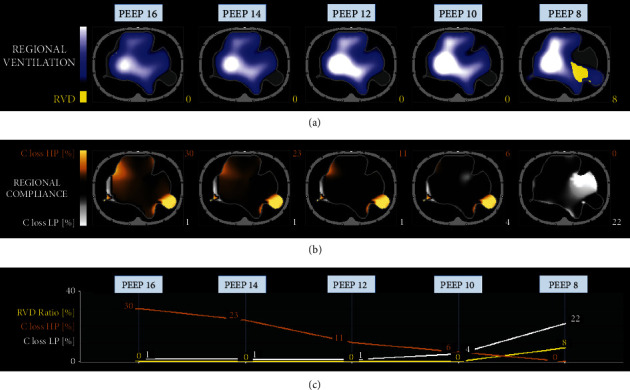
Electrical impedance tomography decremental positive end-expiratory pressure (PEEP) trial during the first extracorporeal membrane oxygenation support. In (a), the regional distribution of tidal ventilation at different PEEP values is shown by the blue–white gradient area, while the yellow area represents regional ventilation delay (RVD), whose percentage is indicated by the yellow number. In (b), regional compliance (C) is analyzed at different PEEP values. Compliance loss secondary to high PEEP (HP) is represented by the orange area (i.e., lung overdistension), while compliance loss due to low PEEP (LP) is represented by the white area (i.e., lung collapse). The percentage amounts of compliance loss associated with HP and LP are quantified by the orange and white numbers, respectively. In (c), RVD and compliance loss due to HP and LP at the different values of PEEP are plotted in order to facilitate the selection of the patient's best PEEP. This electrical impedance analysis shows the extreme heterogeneity of ventilation distribution, reflecting the severe and diffuse lung parenchyma abnormalities, documented also by chest computed tomography.
